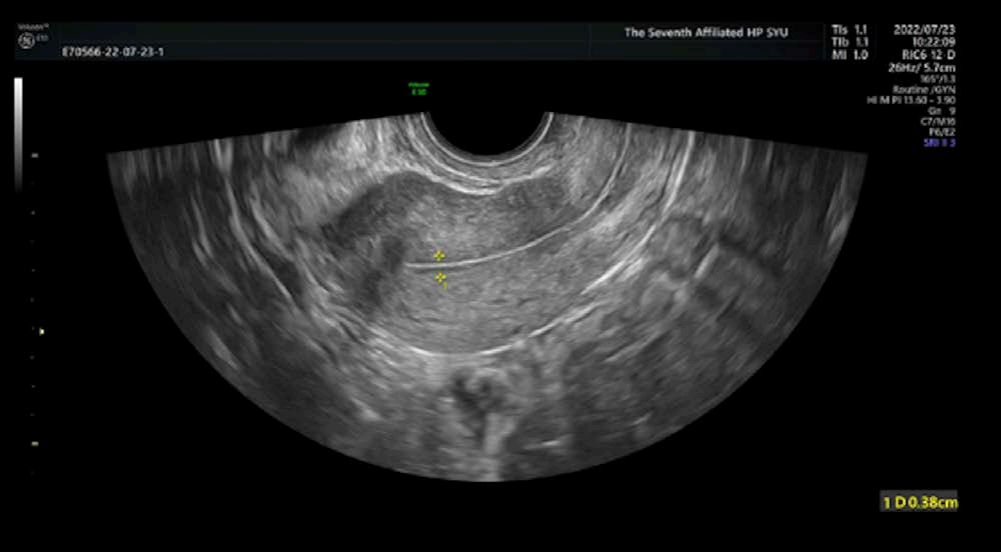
Endometrial ultrasound image on hCG day. On the day of HCG trigger, the thickness of the endometrium is 3.8 mm, and the echo of the endometrium is uniform, presenting a “three line sign”, which is consistent with the appearance of A-type endometrium.
